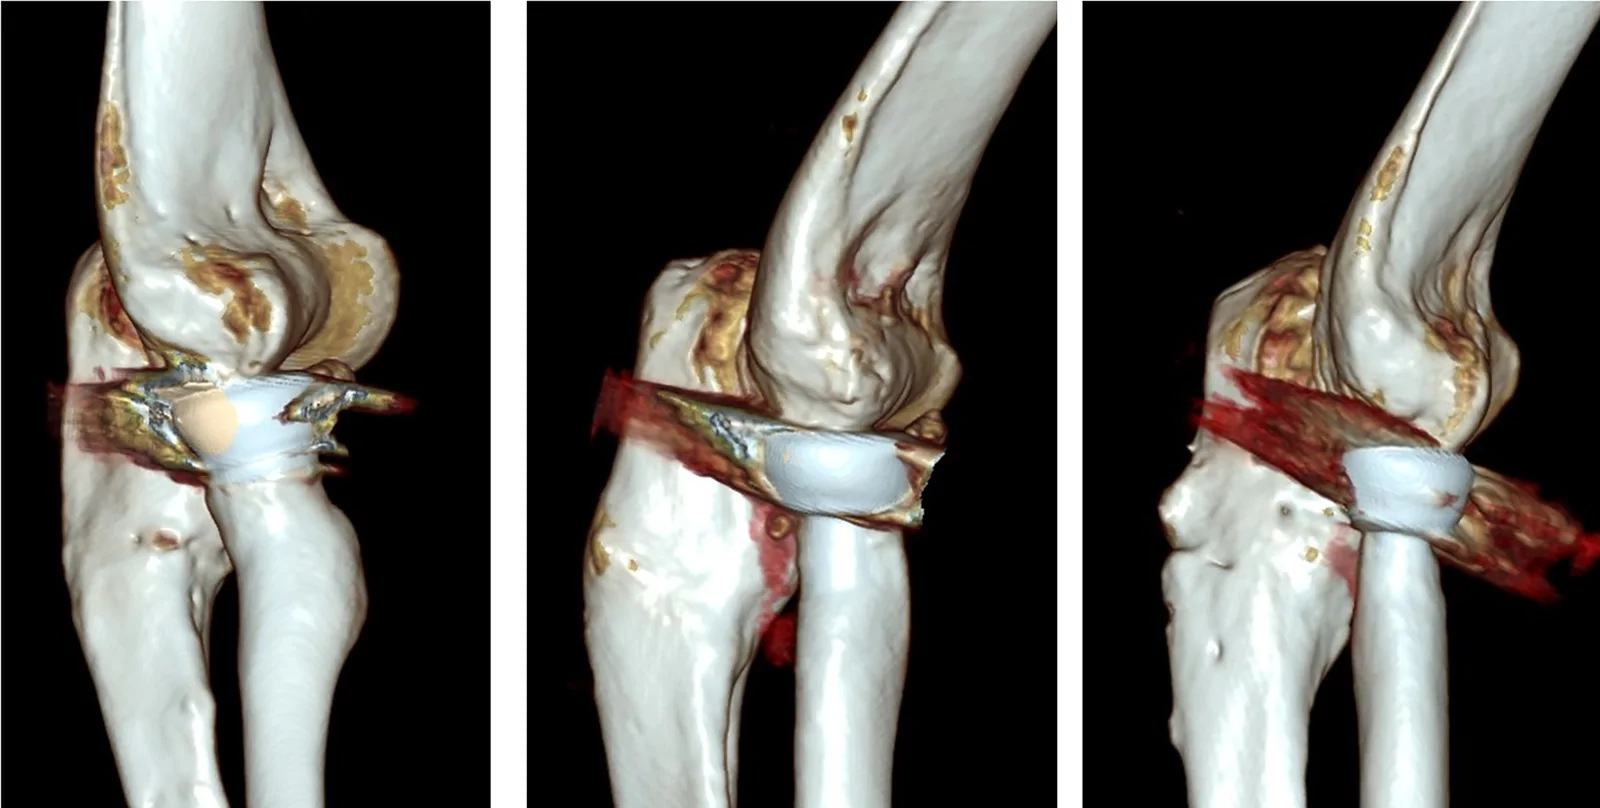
Postoperative CT showing maintained radiocapitellar reduction, prosthesis position, and ulnar osteotomy alignment.
